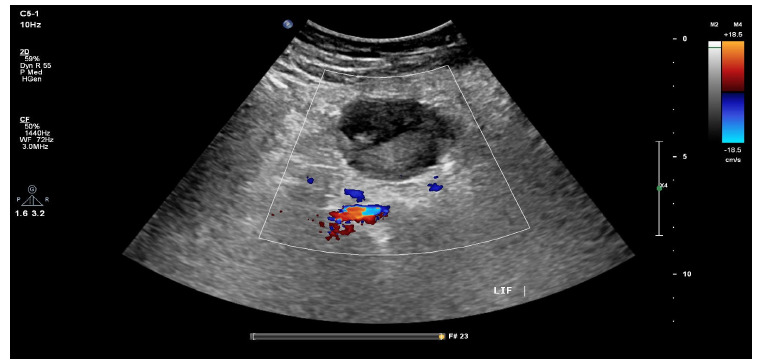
Ultrasound of the left iliac fossa demonstrating a heterogenous lesion measuring 5 cm × 3.5 cm × 4 cm with no internal vascularity.
